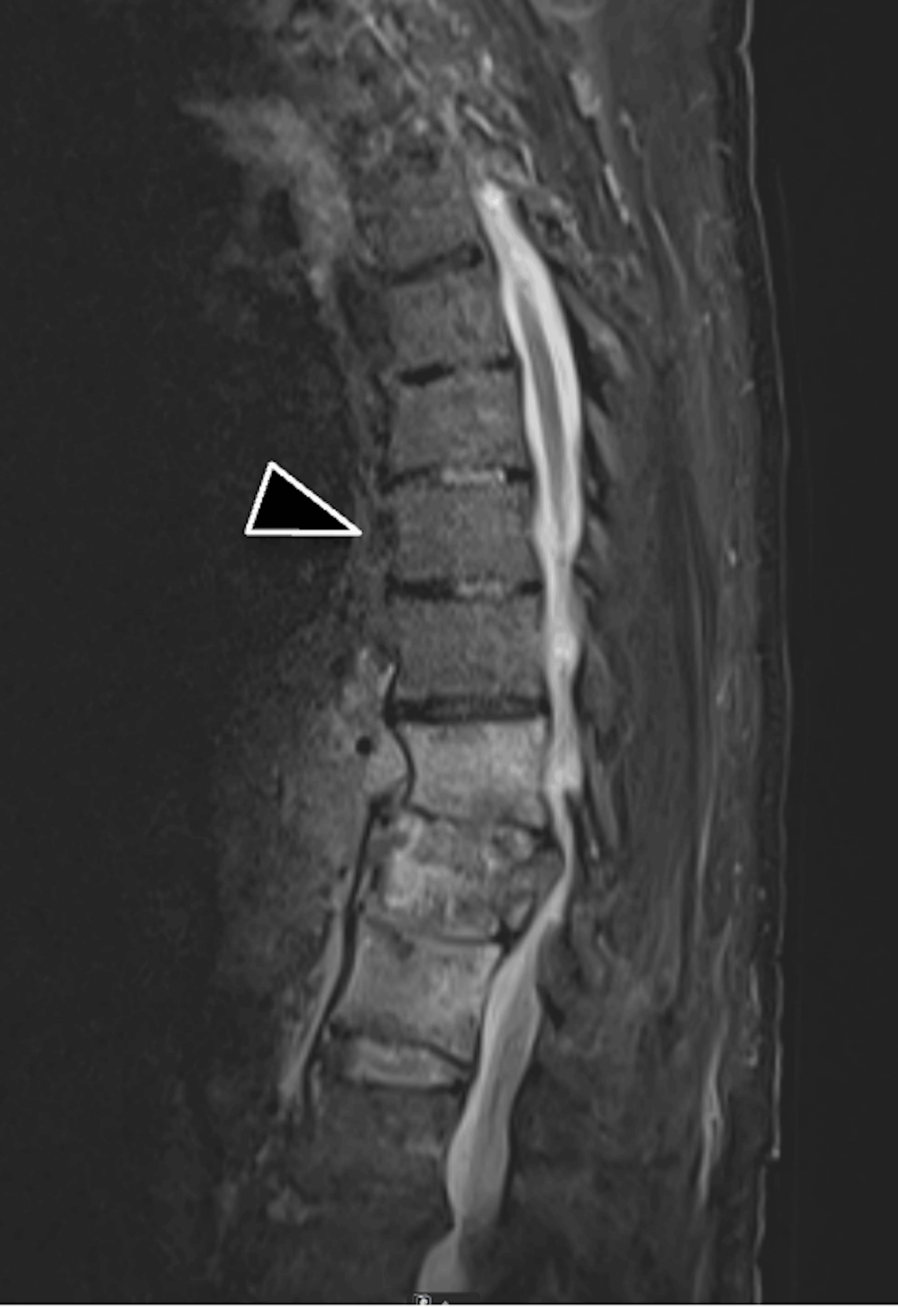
Preoperative T2W/STIR MRI thoracic spine. Preoperative T2-weighted (T2W)/Short Tau Inversion Recovery (STIR) MRI thoracic spine of this 79-year-old man, acquired two days preoperatively, demonstrating a T9 vertebral body (black arrowhead) with no evidence of fracture or injury.
